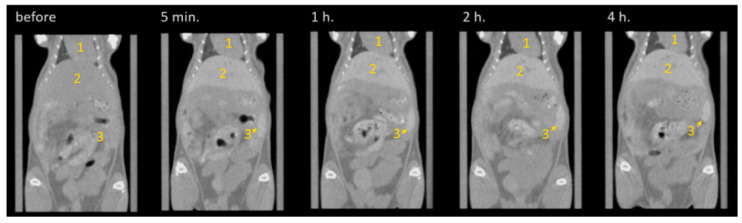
CT images taken before (left) and with different intervals after injection of PEG-capped GdF_3_:Tb^3+^ NPs. Different organs are denoted in the images: 1—heart, 2—liver, 3—spleen; the kidney is not visible within the selected 2D slice. All the rest of the structures observed in the abdominal area represent the organs of the gastrointestinal tract and bladder, which provide notable contrast also before NPs’ administration (see left panel—“before”).
